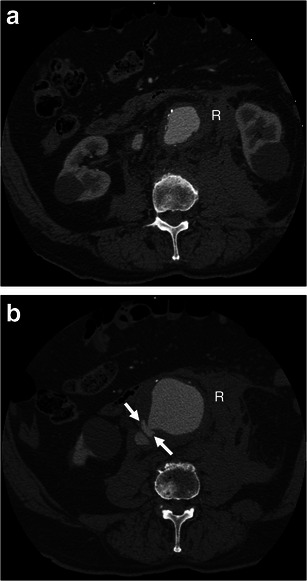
Aortocaval fistula. a Axial arterial phase enhanced CT shows simultaneous enhancement of the AAA and IVC in an 83-year-old man with AAA rupture and retroperitoneal haematoma (*R*). b Axial enhanced CT of the same patient at a lower level demonstrates active contrast extravasation (*white arrows*) from the aortic aneurysm to the IVC with loss of normal fat planes between the structures. Retroperitoneal haematoma (*R*) can also be seen
